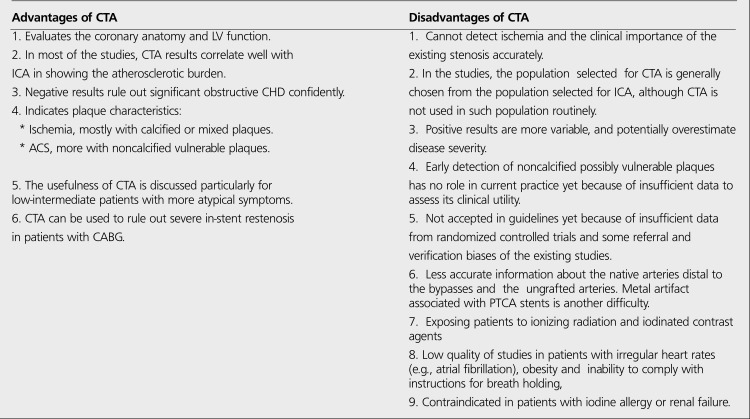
Advantages and disadvantages of CTA used in the diagnosis of CHD (78,85,86,87)
